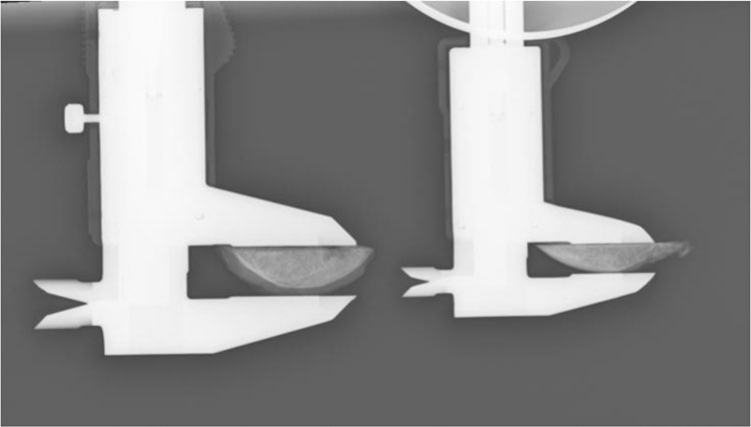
Radiographic setup for measurement of cartilage thickness.
